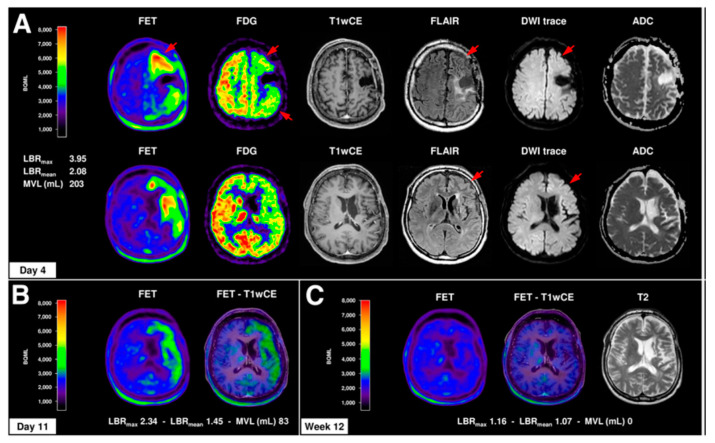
Cortical amino acid uptake in O-(2-^18^F-fluoroethyl)-L-tyrosine (FET) PET in the course of a prolonged postictal episode. A 44-year-old man with clinically stable anaplastic astrocytoma WHO III without any evidence of a residual tumor over years presented with tonic-clonic seizures followed by severe and prolonged postictal symptoms (global aphasia, right-sided hemiplegia, and hemineglect) over an 8-week period. (A) MRI (day 1) and FET PET (day 4) showed distinct increased and extended cortical FET uptake of the left hemisphere (LBR_max_, 3.95; LBR_mean_, 2.08) with frontal and temporal accentuation, corresponding to slight cortical vasogenic and cytotoxic edema (T2/FLAIR, DWI/ADC) without contrast enhancement (T1wCE). EEG monitoring, ^18^F-FDG PET (glucose hypometabolism, red arrows), and 99mTc-HMPAO SPECT (showing hypoperfusion), however, revealed no evidence of status epilepticus. (B) For FET PET 11 days after symptom onset and 7 days after the first FET PET, slight regression of cortical FET uptake (LBR_max_, 2.34; LBR_mean_, 1.45) was observed. (C) The patient slowly recovered within 8 weeks after seizure onset. FET PET and MRI 12 weeks after symptom onset demonstrated complete recovery of cortical FET uptake and brain edema; only residual cortical atrophy in T1 and T2/FLAIR sequences remained. (Reproduced with permission from Figure 3 of Hutterer et al. [30]). LBR: lesion-to-brain ratio.
